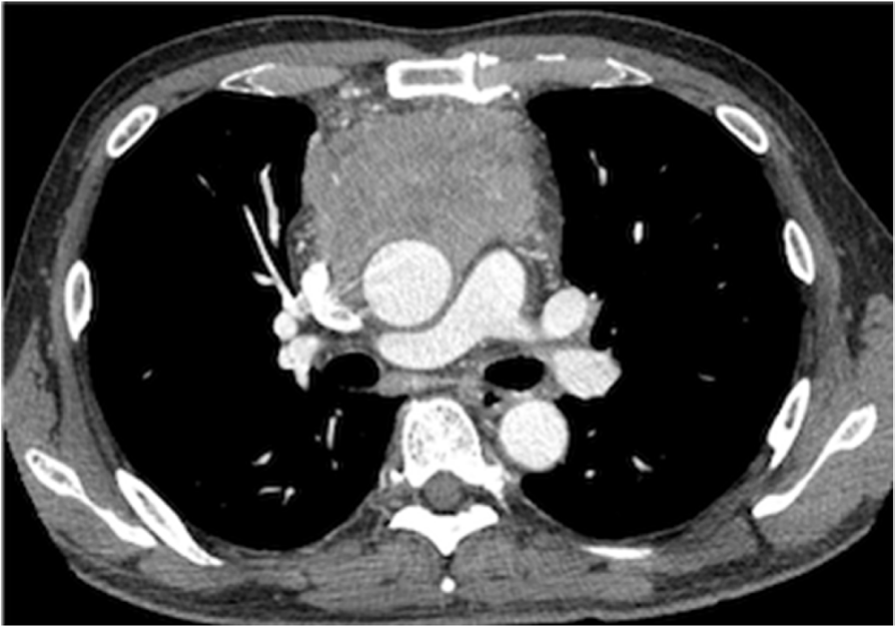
Initial contrast-enhanced chest CT image showing an irregular 8.4 × 6.0 cm mass in the anterior mediastinum. The mass abuts the ascending aorta and compresses the SVC, suggesting invasion
